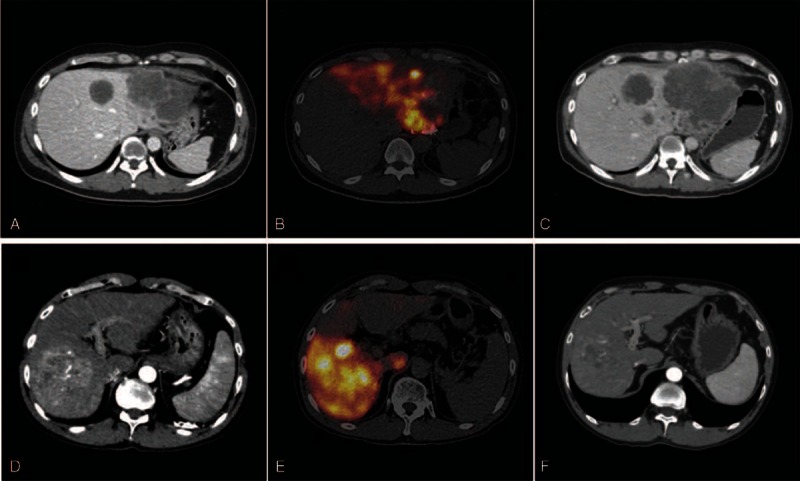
Representative cases. A 37-year-old male patient with hepatocellular carcinoma presented with a large mass in the left lobe with central necrosis on pre-SIRT contrast-enhanced CT (A). SIRT was performed and 2.3 GBq ^90^Y-microsphere was injected through the left hepatic artery. ^90^Y-microsphere PET/CT showed poor delivery of ^90^Y-microsphere to the central portion of the tumor (B). Tumor-absorbed dose was evaluated as 141.3 Gy. Post-SIRT CT showed enlargement of the tumor (C), and PFS was 21 days. Another 55-year-old male patient with hepatocellular carcinoma presented with a large mass in the right lobe on pre-SIRT CT (D). SIRT was performed through the right hepatic artery and 3.1 GBq ^90^Y-microsphere was injected (E). Tumor-absorbed dose was evaluated as 205.0 Gy. Post-SIRT CT showed shrinkage of the tumor (F) and PFS was 126 days.
